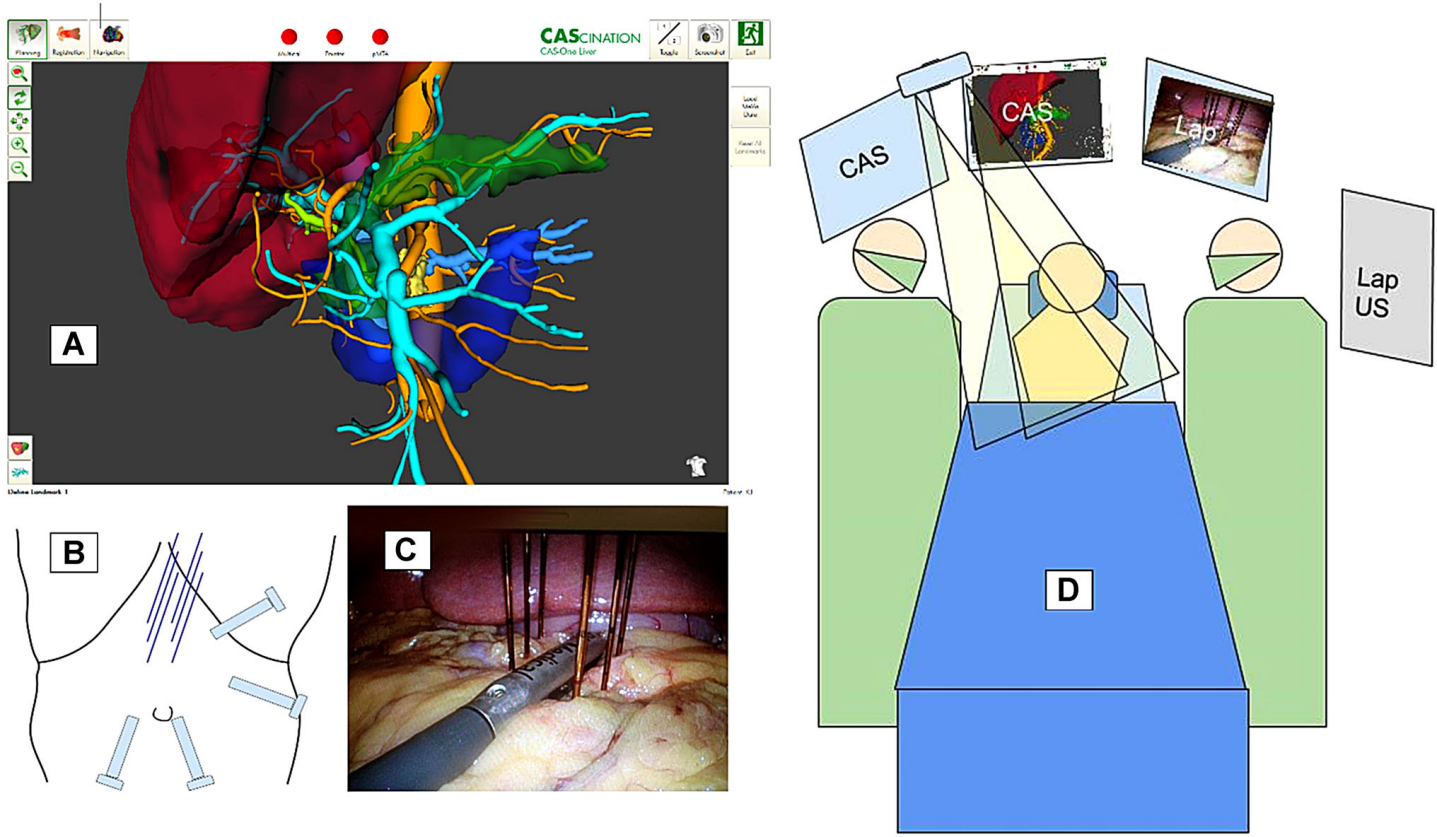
Patient setup with CAS-One on the patients right with twin infrared cameras and laparoscopic screen and ultrasound screen on the left. A On-screen image of rendered abdominal structures in the MeVis model. B Laparoscopic port placements and site of IRE needle entries. C Laparoscopic view of laparoscopic ultrasound device aligned over the superior mesenteric artery and IRE needles inserted in a 2 × 3 fashion to surround the tumor. D Setup of surgeons and monitors
